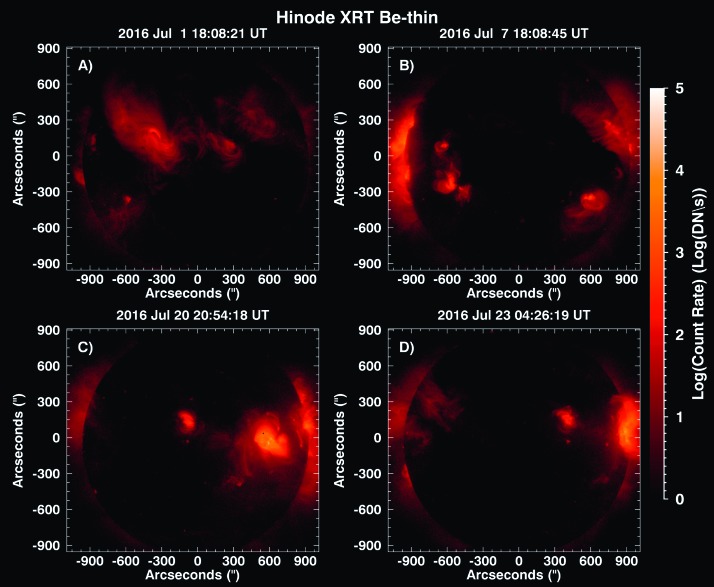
(A) Log-scaled count rate *Hinode*/XRT Be-thin full-Sun images near the time of the MinXSS-1 observed QS. (B), (C), and (D) Pre-flare times for the C2.7, M1.2 and M5.0 flares, respectively. The XRT images provide information on the spatial distribution of the soft X-ray emitting plasma, since MinXSS measurements are integrated across the entire FOV.
